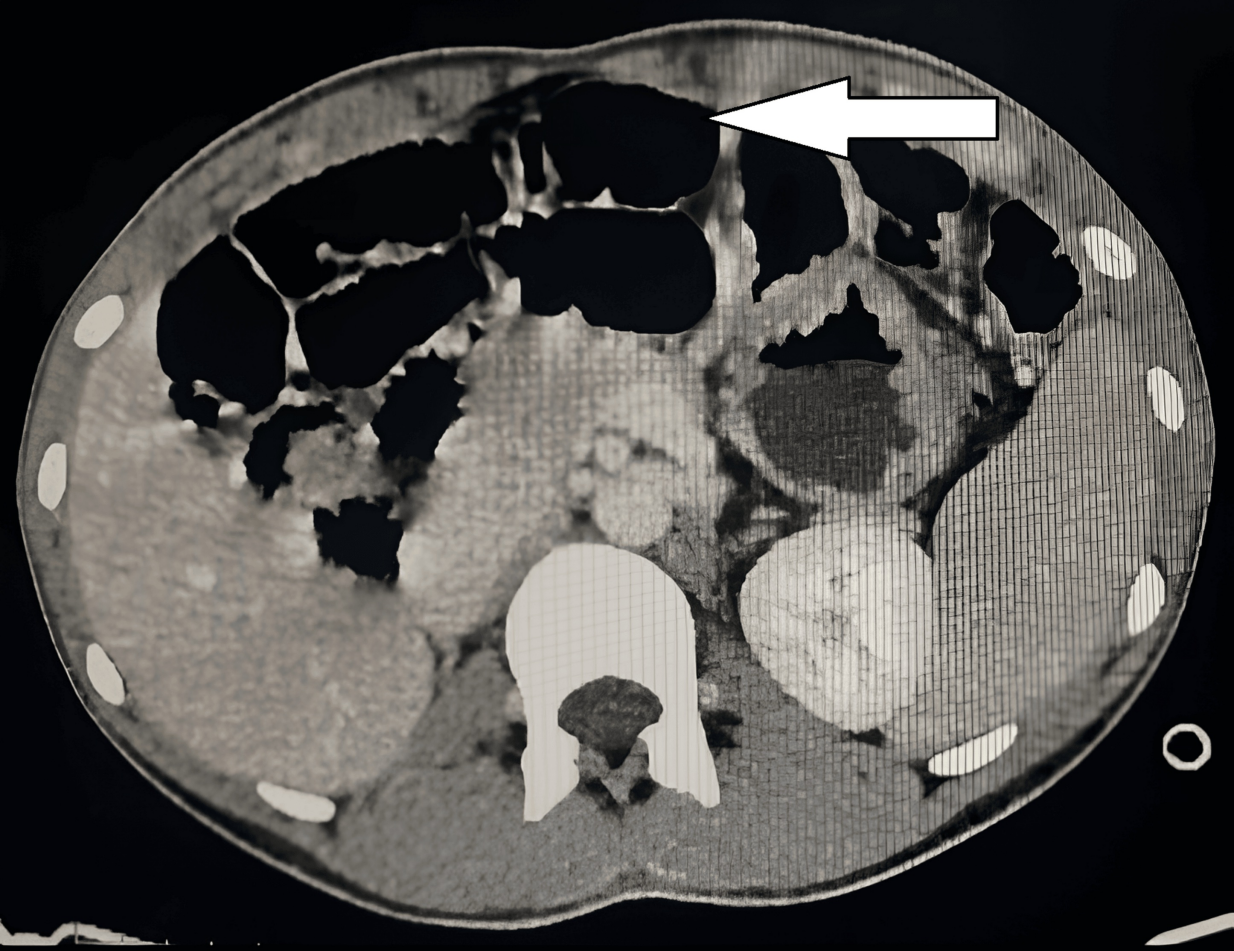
CECT of the abdomen (axial view) The arrow indicates the dilated bowel loop. CECT: contrast-enhanced computed tomography
